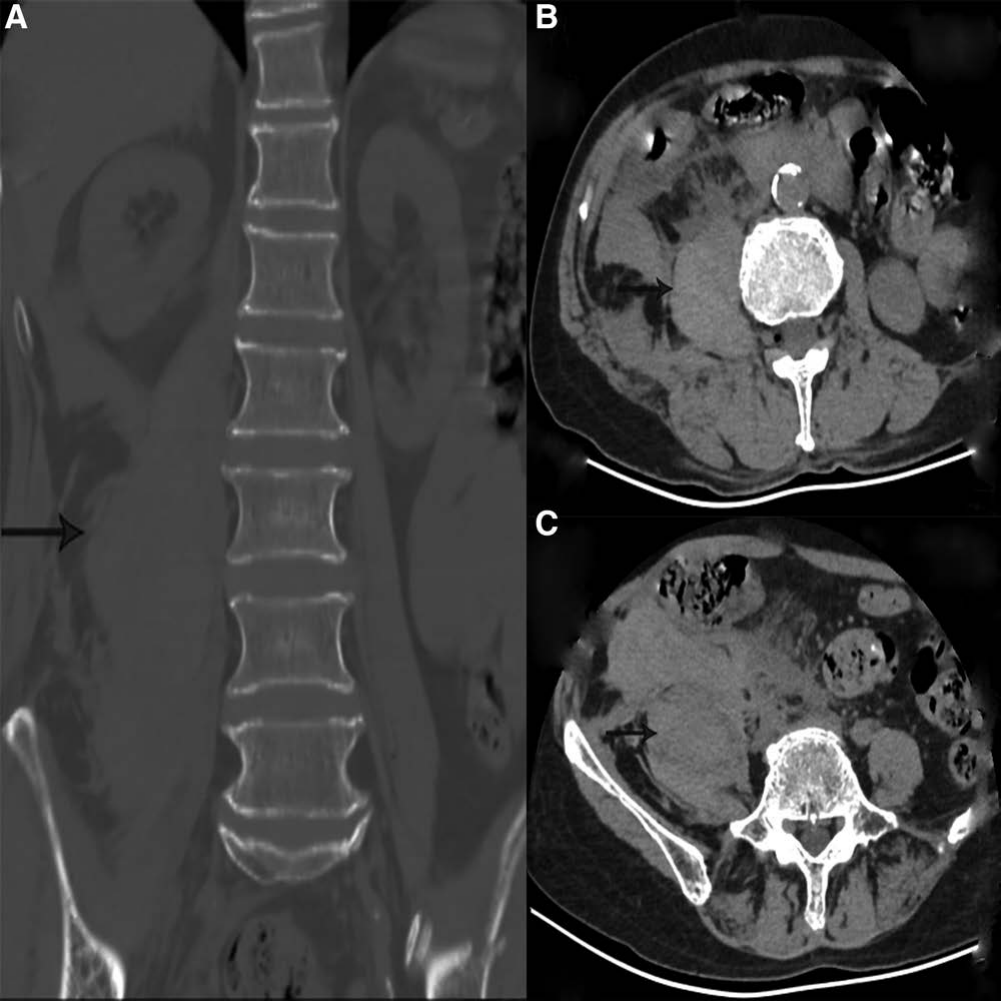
Plain CT image of the lumbar intervertebral disc obtained about 7 h after the treatment of small needle scalpel. (a) Coronal view; (b) horizontal view at the L2 level; (c) horizontal view at the L5 level. CT = computed tomography.
